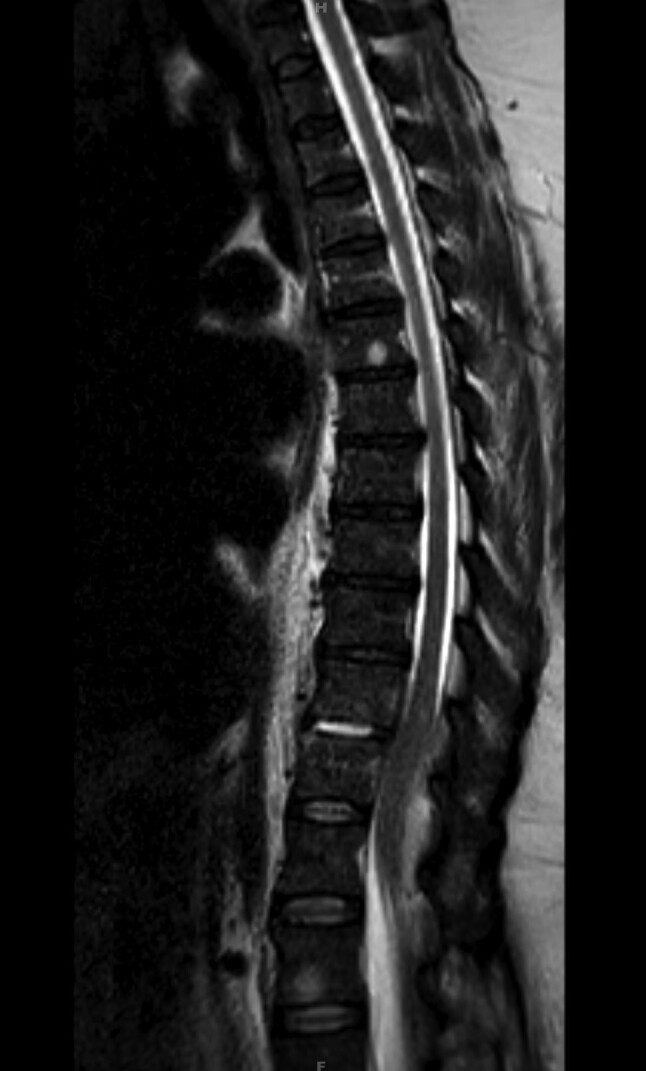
Sagittal view of contrast-enhanced magnetic resonance imaging of thoracic and lumbar spine shows discitis at the T10–T11 disc space level. Abnormal meningeal enhancement is noted at T10–T12, causing some spinal cord compression.
